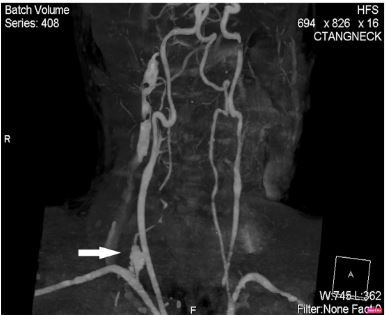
Computed tomography angiogram showing an aneurysm (arrow) at the right vertebral artery origin with poor opacification of the remainder of the vessel.
